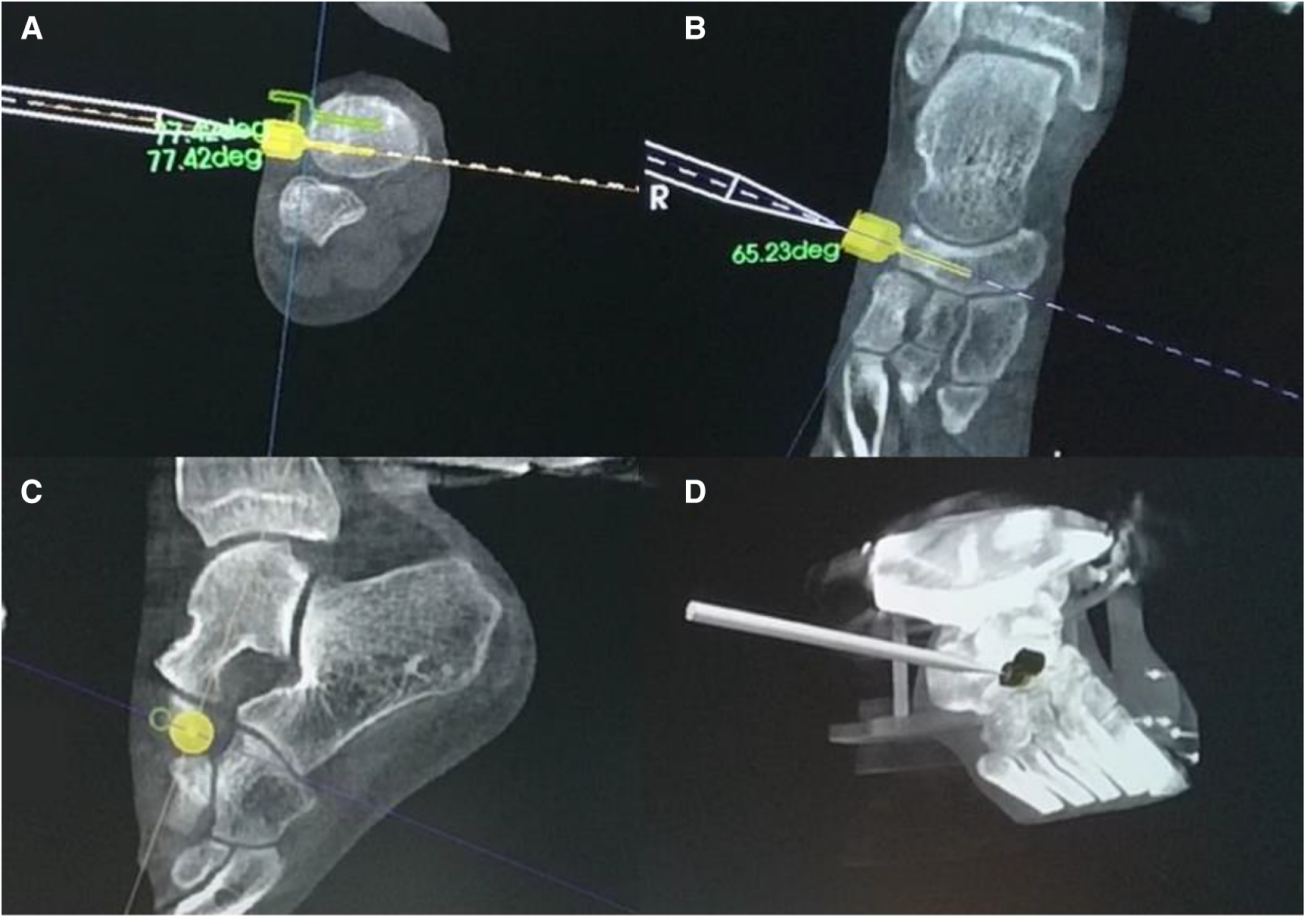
TINAVI intelligent orthopedic robot navigated during the operation and calculated the screw placement trajectory according to three-dimensional CT. (A) CT axial view of the right foot; (B) CT coronal view of the right foot; (C) CT sagittal view of the right foot; (D) 3D CT reconstruction of the right foot.
